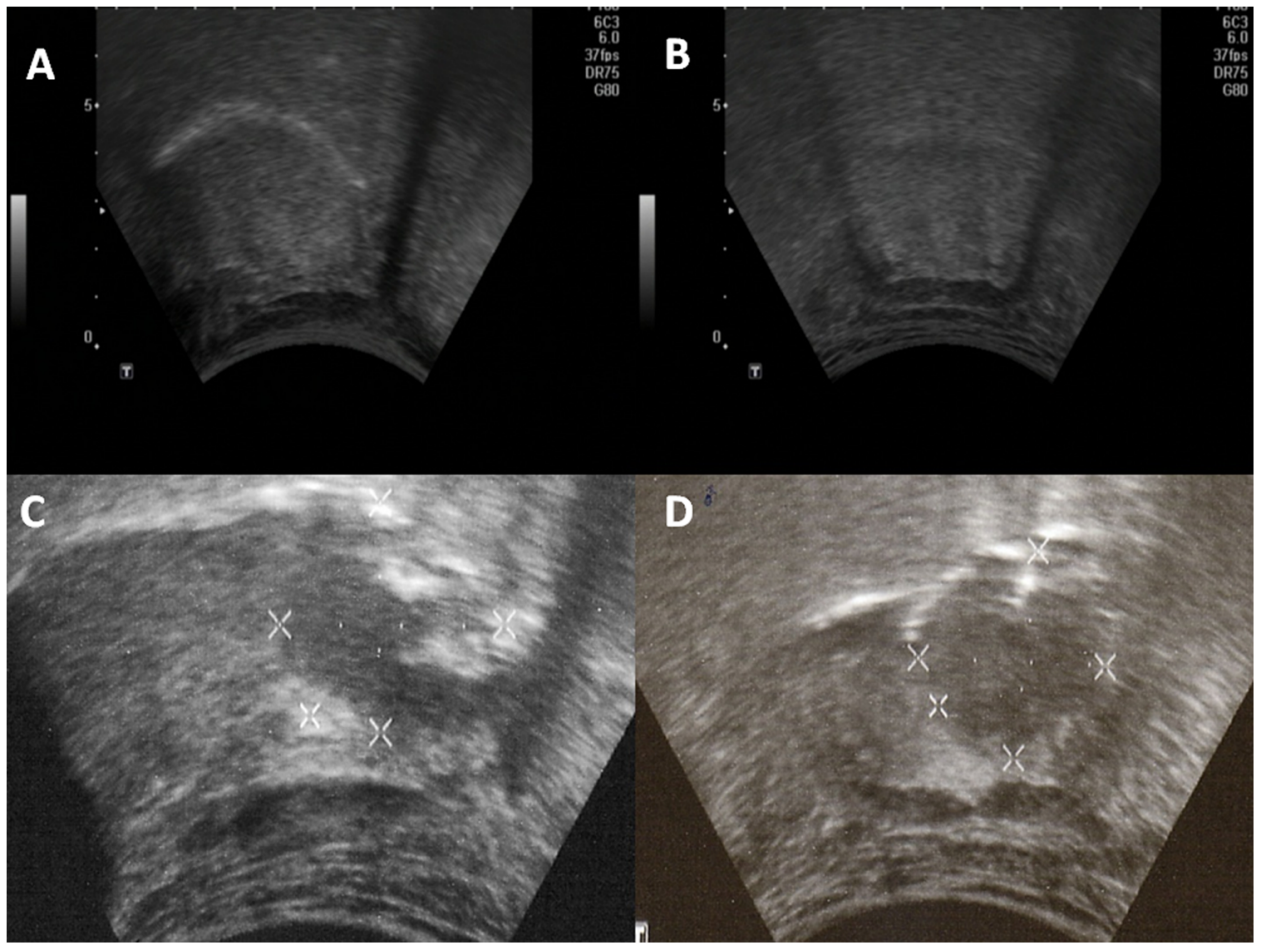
The transcervical ultrasound appearance of the normal BOT is shown in parasagittal (A) and coronal views (B). A BOT tumor is shown in parasagittal (C) and coronal views (D). A large endophytic portion as well as a superficial mucosal component are observed in both views. On coronal view, the relationship with midline is appreciated. The distance between each calibration bar is 10 mm.
